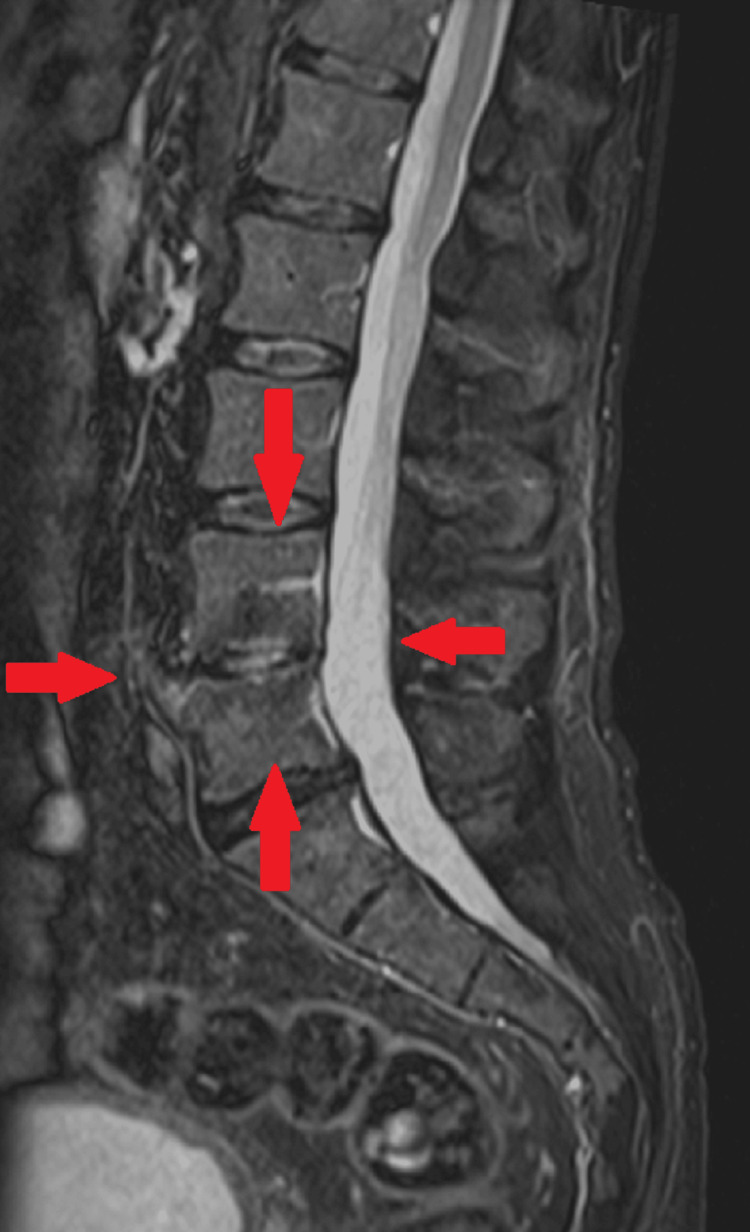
Follow-up (eight weeks) lumbosacral MRI (STIR, sagittal) showing resolution of the epidural collection STIR, short tau inversion recovery
